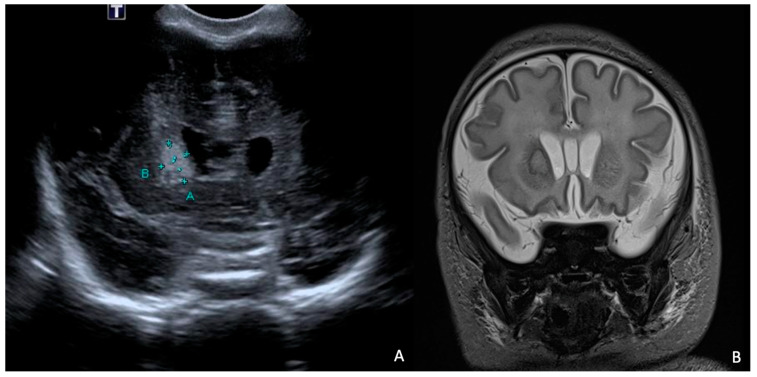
HUS (A) and brain MRI in T2 sequence (B): abscess of approximately 1 cm in diameter (delimited by the letters A and B in (A)) at level of right caudate nucleus in context compatible with hypoxic–ischemic encephalopathy. MRI in T2 sequence showed evidence of rim and hyperintense core.
